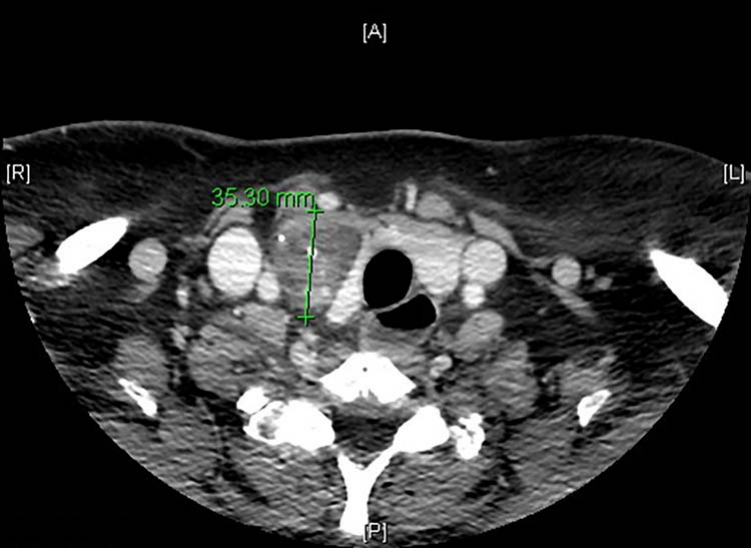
Axial CT scan image showing a right thyroid nodular lesion, discovered incidentally.
